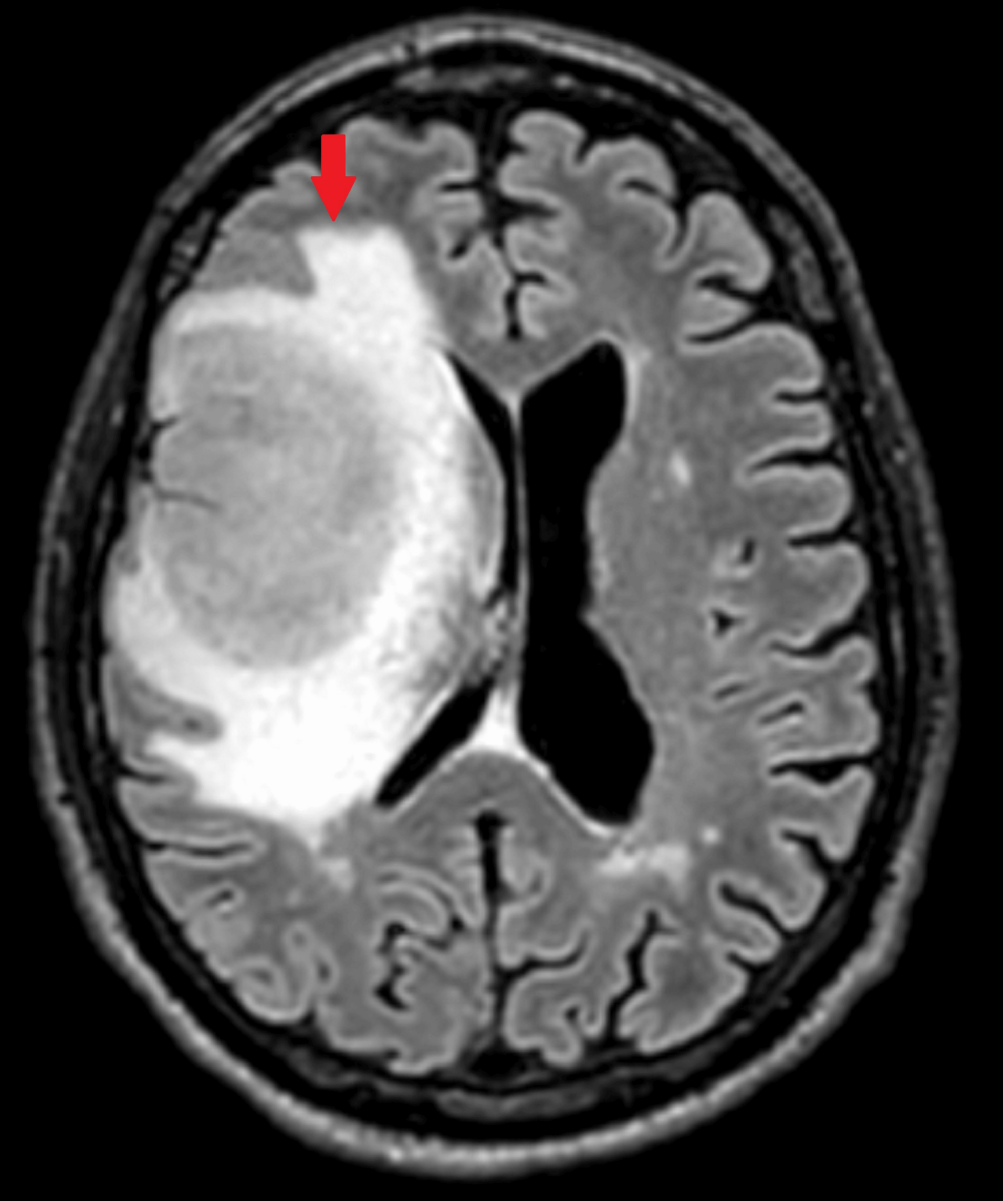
Transverse section of MRI shows a brain mass
